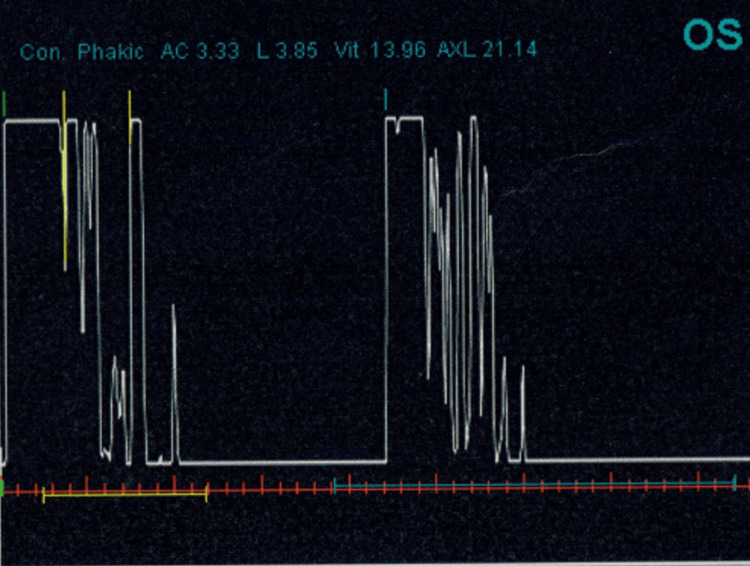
Ultrasound A-scan demonstrating normal globe structure in the left eye
